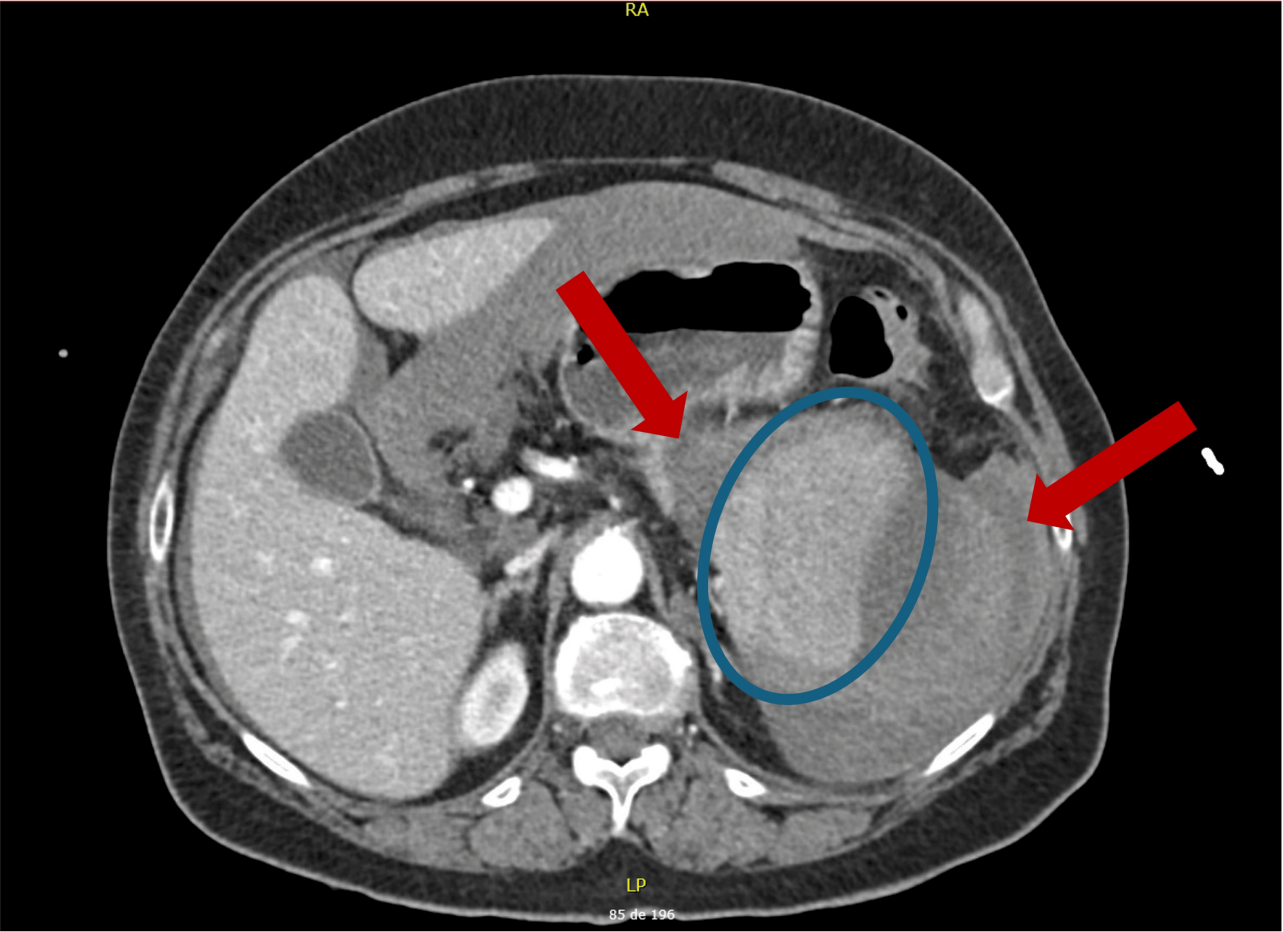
Axial contrast-enhanced CT of the abdomen and pelvis blue circle: large subcapsular hematoma; red arrows: active contrast extravasation
